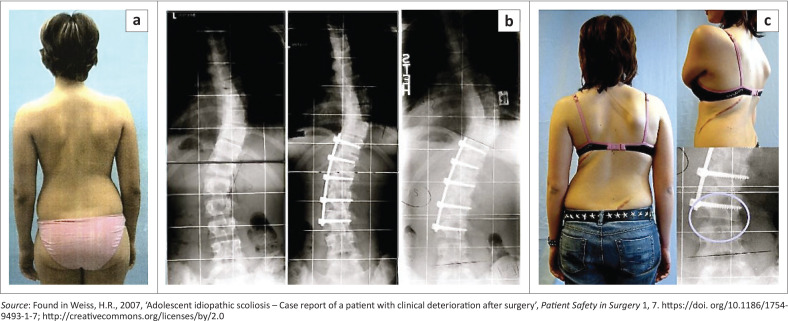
(a) 14-year-old, almost fully mature girl with AIS and balanced appearance, (b) native X-ray before and after the operation. (c) Appearance after surgery with clear decompensation and radiologically visible wedge deformation of the intervertebral space below the spinal fusion.
